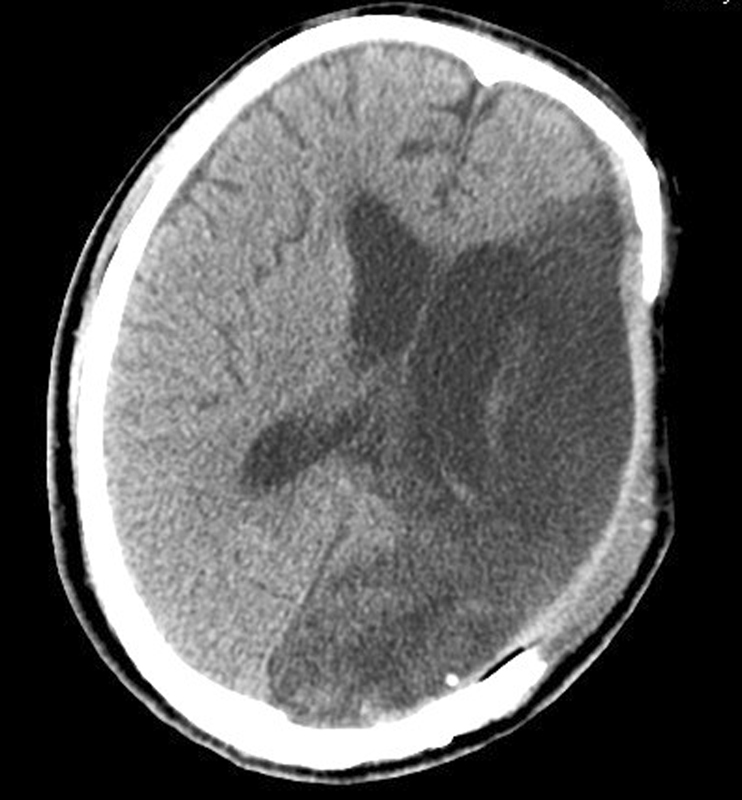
Computed tomography at discharge.
